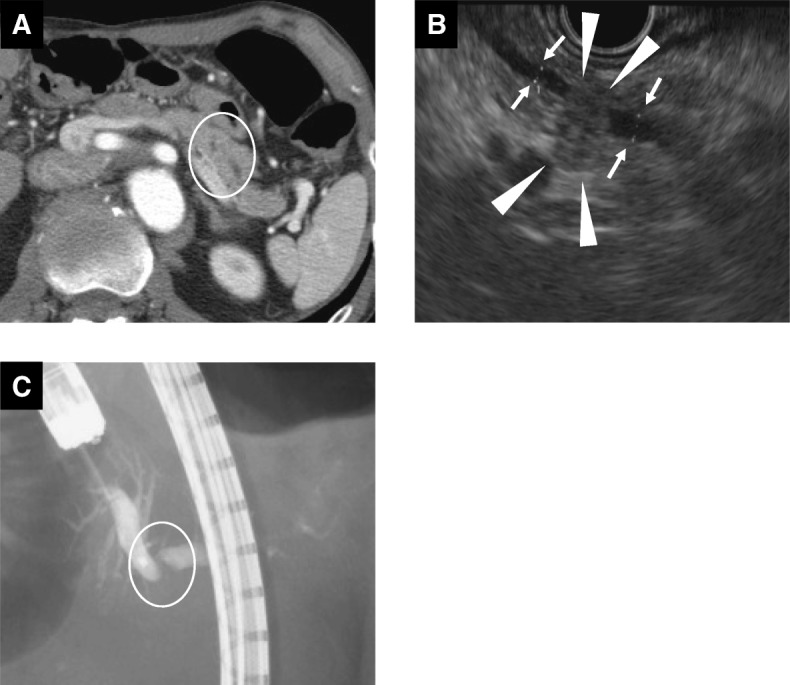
Preoperative imaging findings during the assessment of the resected remnant pancreas lesion 16 years after the initial operation. a Enhanced computed tomography showed a low-density mass at the remnant pancreatic body (circle). b Endoscopic ultrasonography showed an isoechoic and hypovascular mass (7 mm in diameter) protruding into the main pancreatic duct (MPD) of the pancreas body (arrowhead). A slight change in the diameter of the MPD between the proximal side and the distal side of the mass was observed (arrow). The MPD wall adjacent to the mass was ill-defined. c Endoscopic retrograde pancreatography showed a localized narrow segment with irregular tapering (4 mm in length) in the MPD of the remnant pancreatic body (circle)
